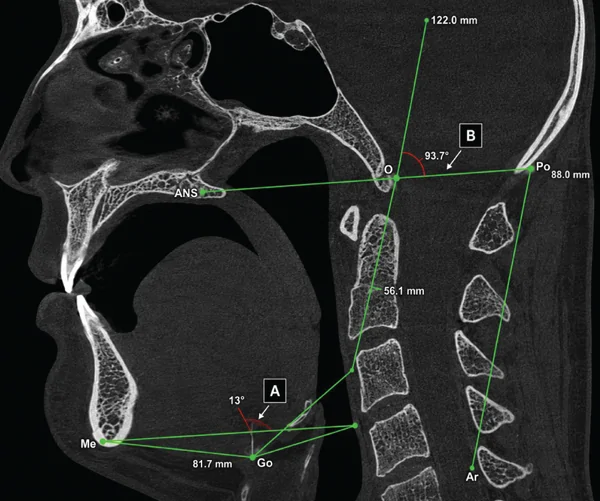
Posttreatment CBCT of Case 2. (A) A hypodivergent hyoid triangle with the hyoid bone placed at C3 with an angulation of 19 degrees (B) Cervical vertebrae alignment.
